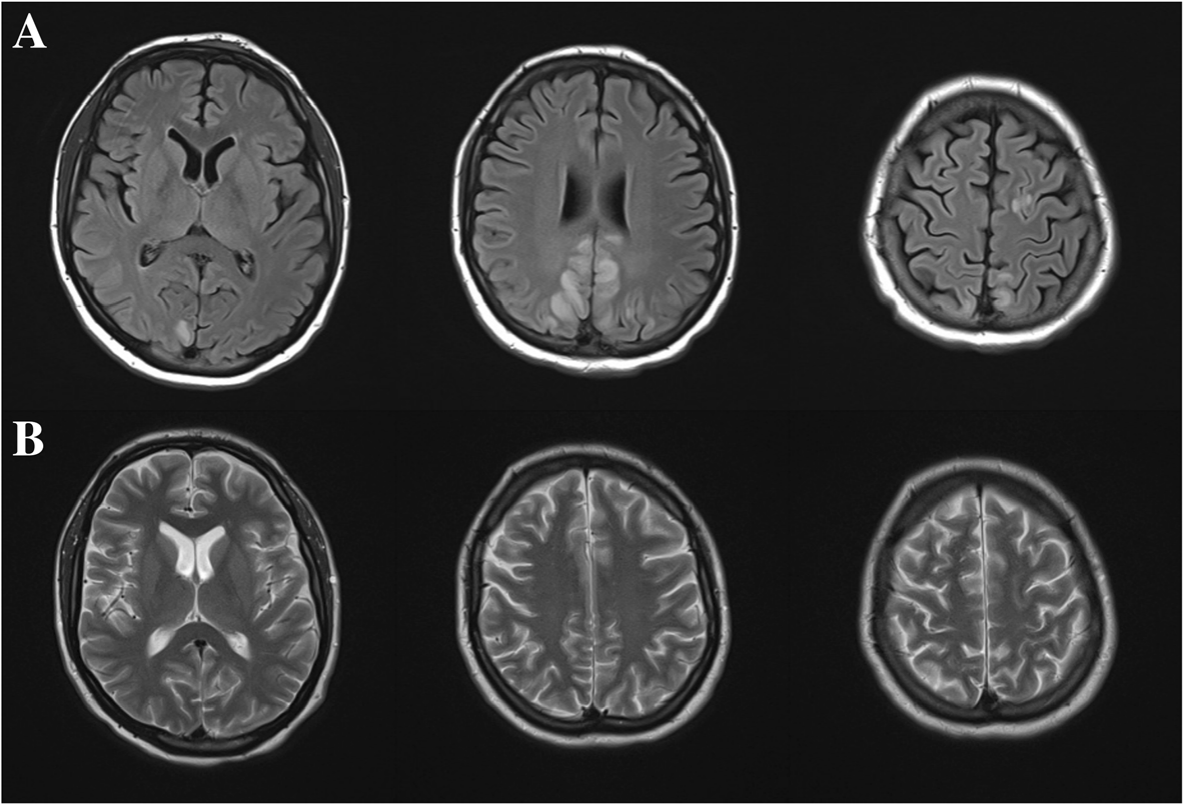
Axial MRI brain of patient. In panel A, there are T2-FLAIR hyperintensities involving the paramedian parietal and occipital lobes, suggesting vasogenic edema. T2-weighted images in panel B were taken three months after discharge and show a resolution of the abnormalities, confirming the diagnosis of PRES.
